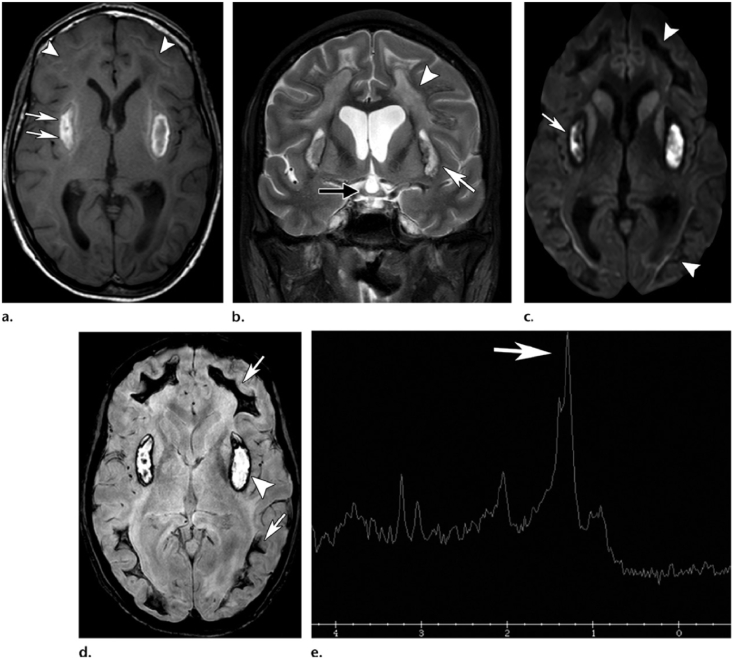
Basal ganglia and putamen involvement in methanol toxicity in MRI or CT scan view.
